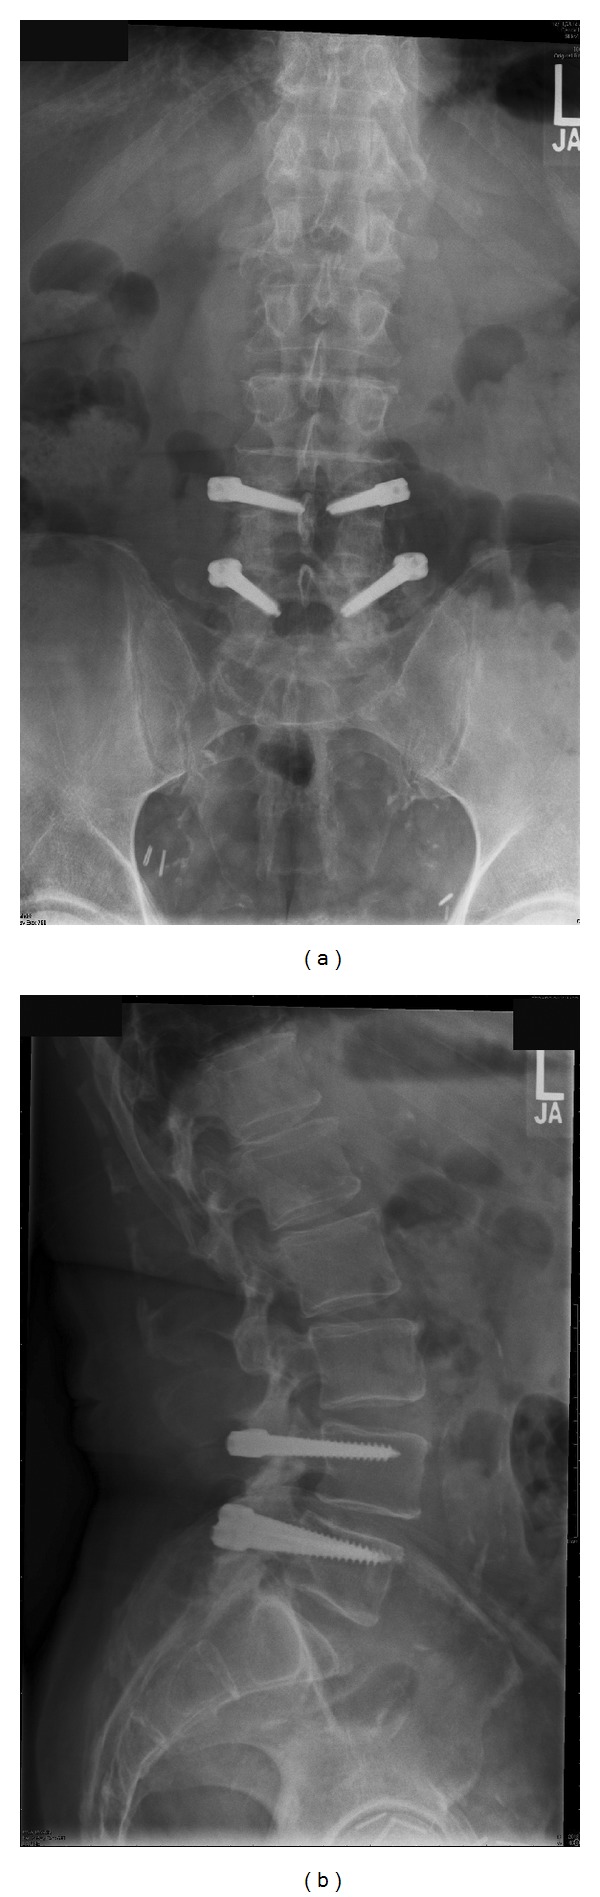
(a) and (b) Postop. anteroposterior and lateral radiographs at 7.5 years postop. showing instrumentation intact and disc height comparable to preop.
